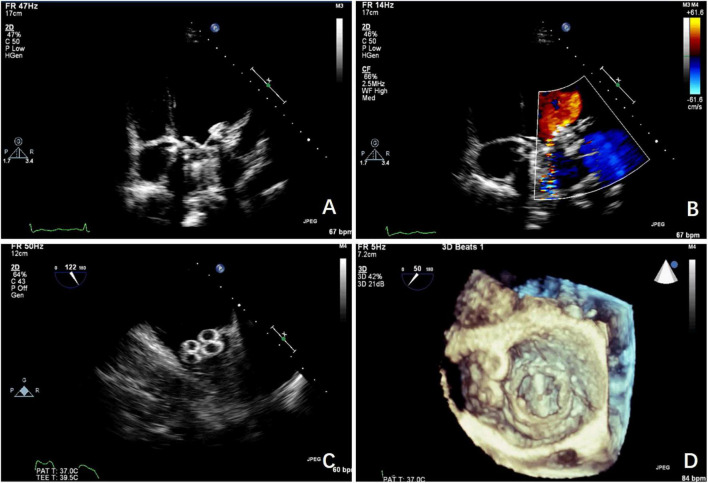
Echocardiograms taken during the follow-up period. (A) TTE shows the mitral PVL after the procedure. (B) TTE shows the mitral PVL closed with the occluder. (C) The mitral PVL is closed with 2 occluders with TEE guidance. (D) The mitral PVL is closed with 2 occluders under 3D TEE guidance.
